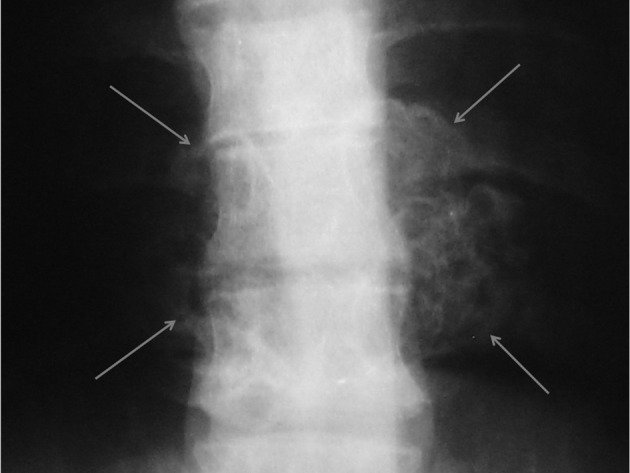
AP plain X-ray of the thoracic spine. A heterogeneous bony mass is seen in the lower thoracic spine.
